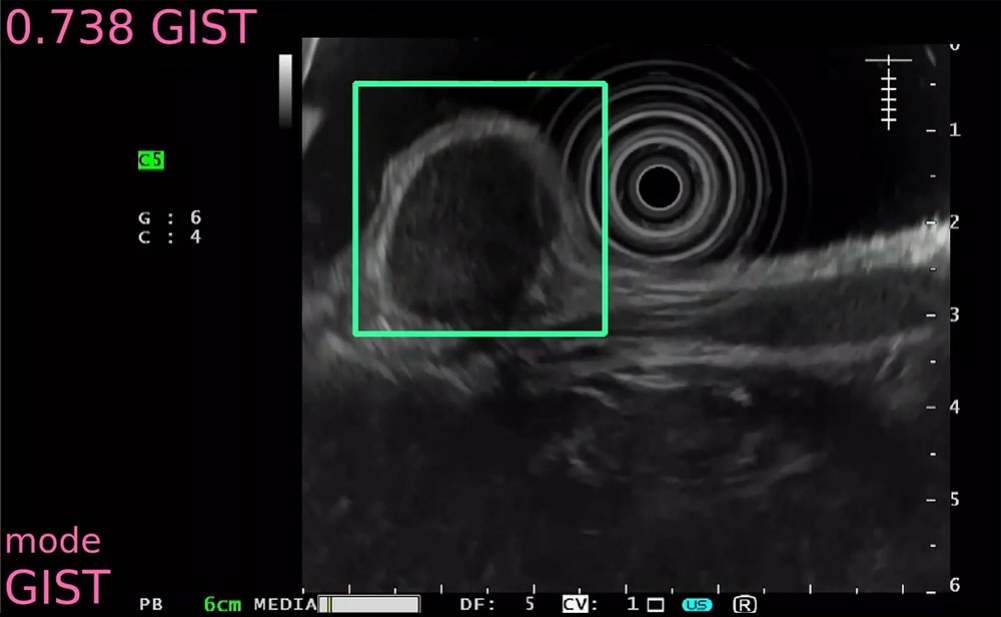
Artificial intelligence image for differential diagnosis of subepithelial lesion. Endoscopic ultrasonography (EUS) image (gastrointestinal stromal tumors, GIST) is used for the differential diagnosis of subepithelial lesions by artificial intelligence. The probability of one EUS image is expressed in the upper left and artificial intelligence diagnoses this lesion as GIST.
